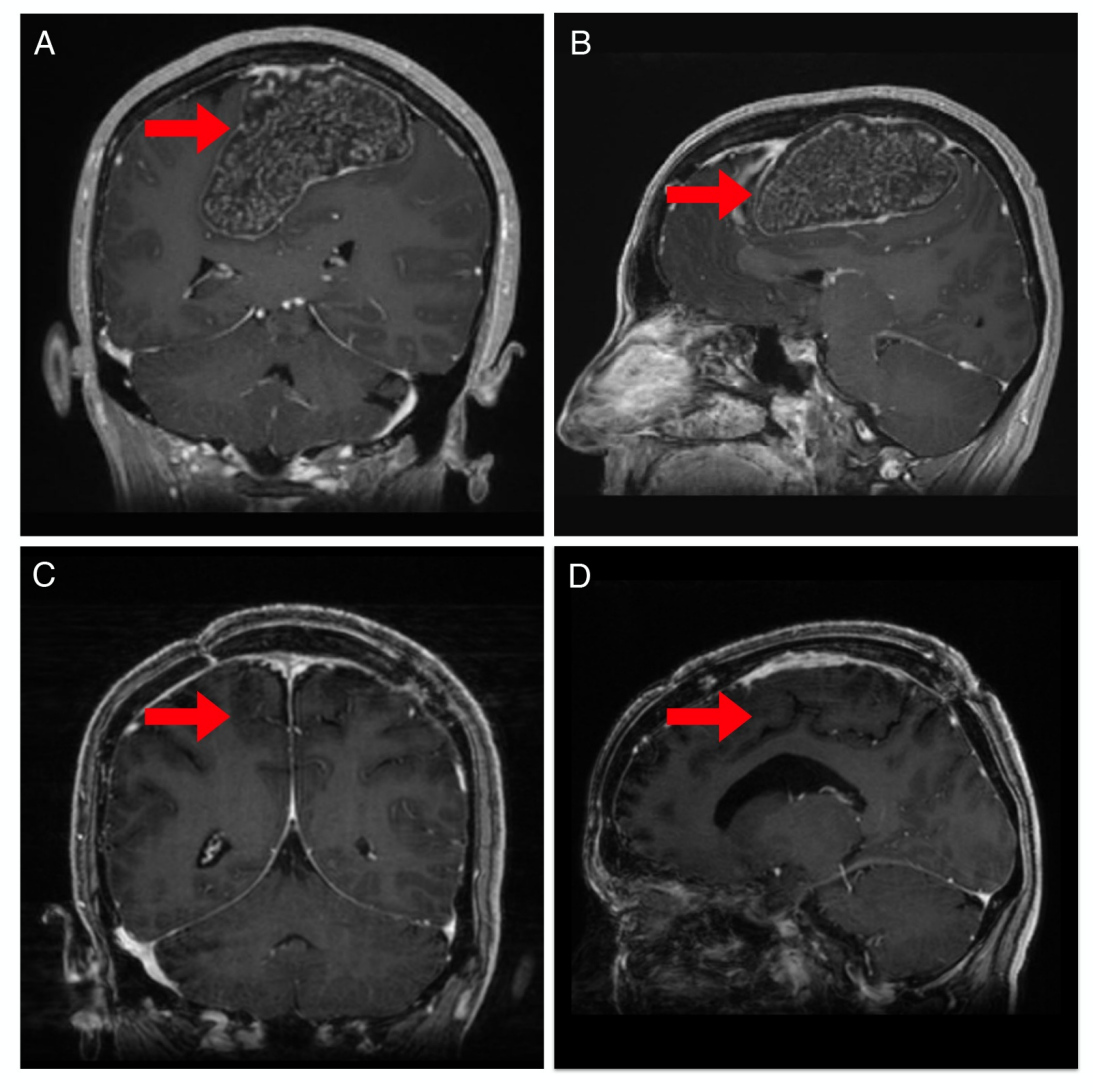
Pre and postoperative magnetic resonance imaging (MRI) Coronal (A) and sagittal (B) MRI imaging (Bravo sequence) revealed an 8.7 x 5.5 x 4.6 cm tumor (red arrow). Coronal (C) and sagittal (D) postoperative MRI imaging (Bravo sequence) showing complete resection of the tumor (red arrow).
